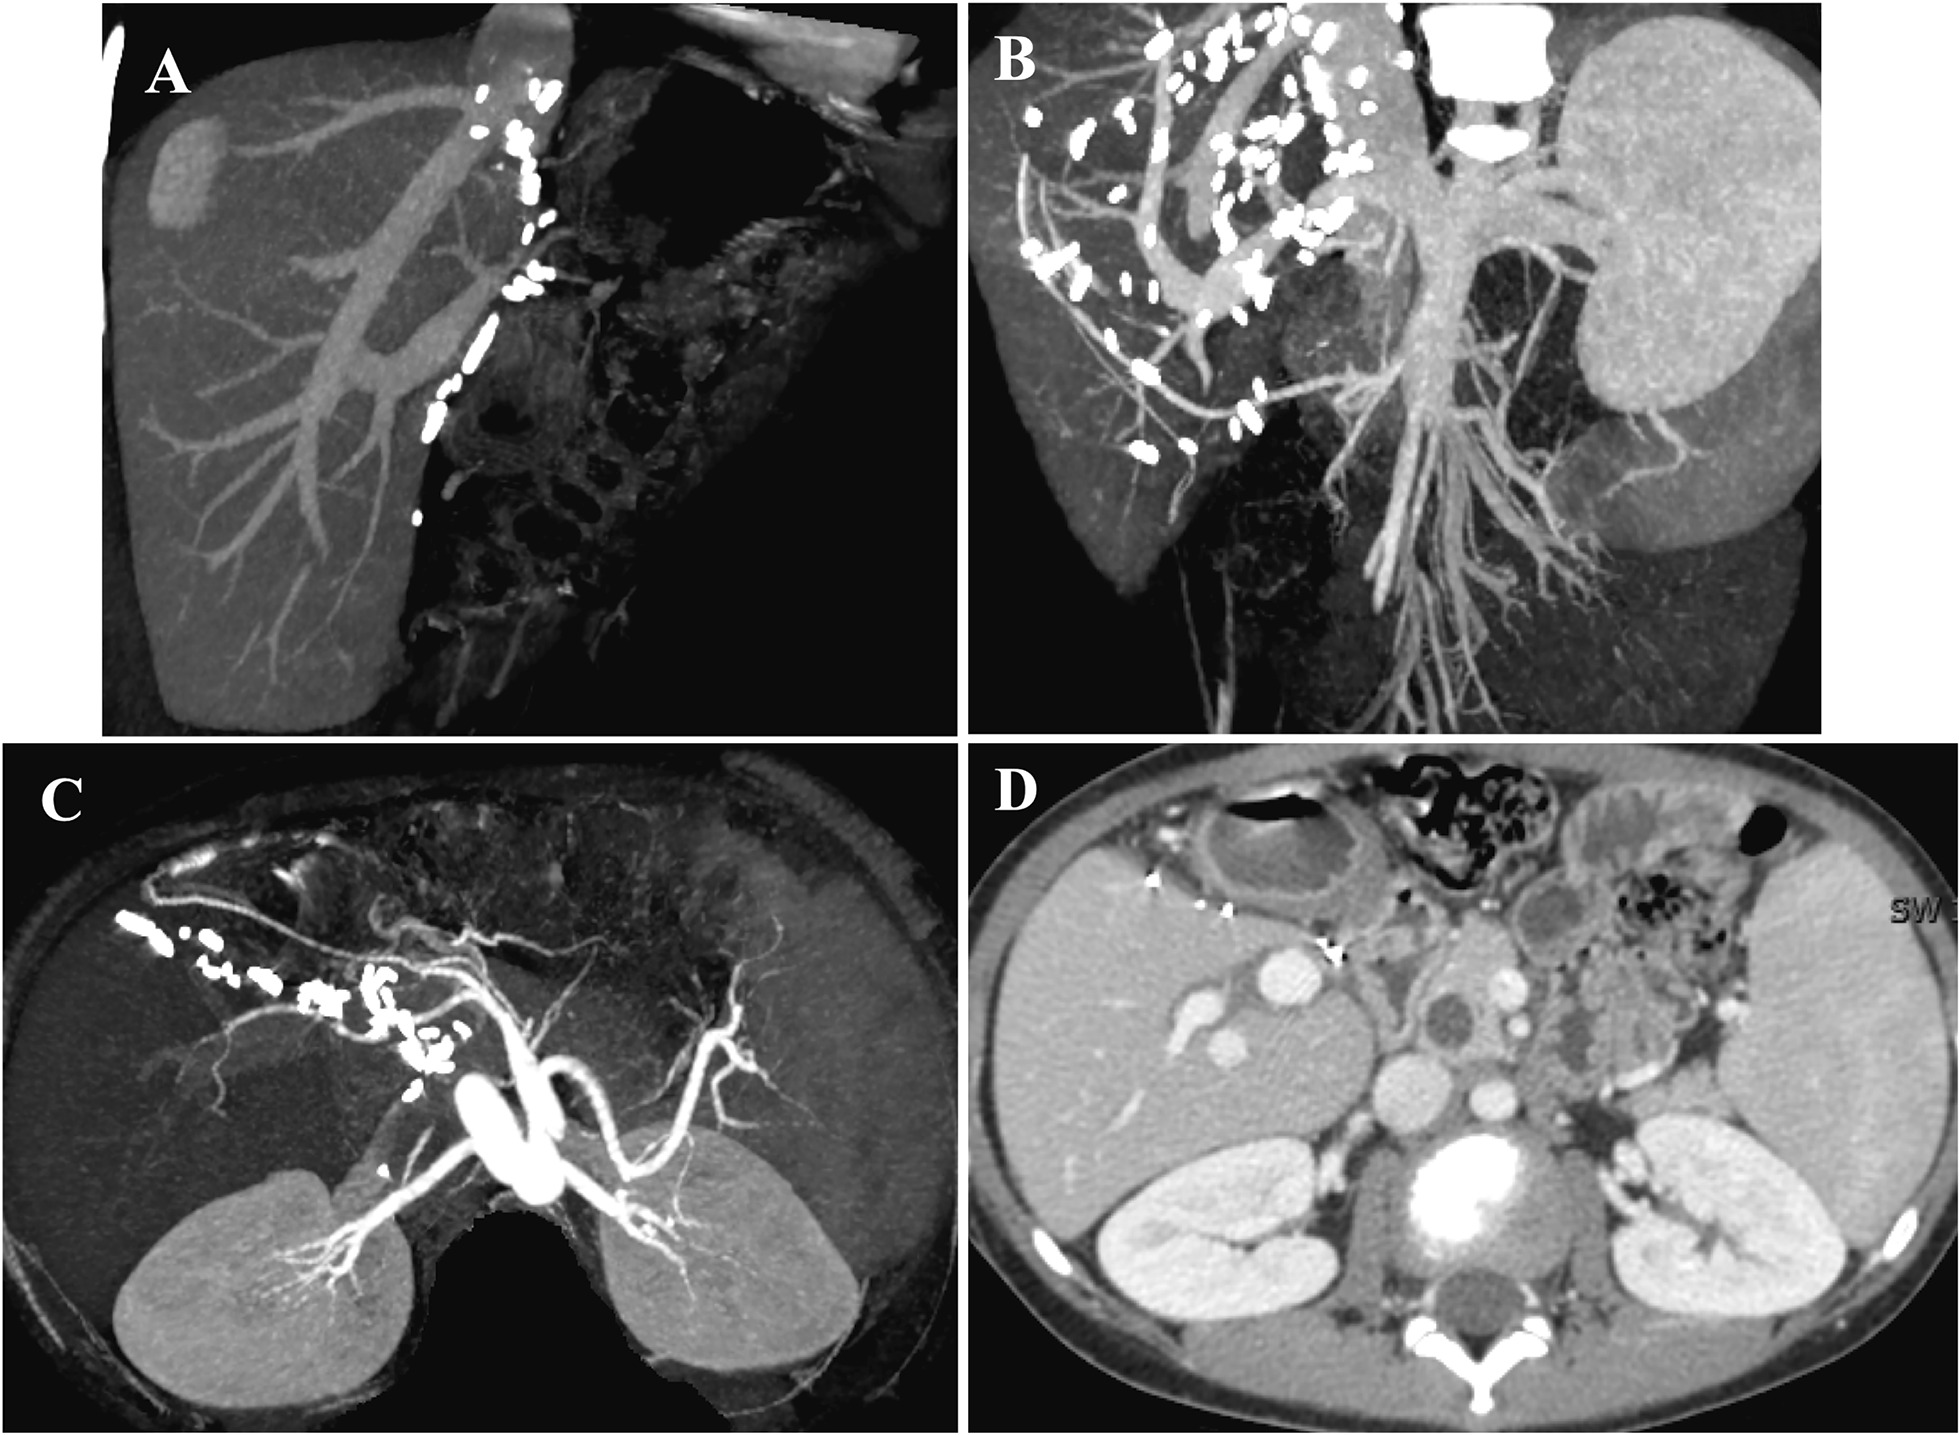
Postoperative imaging of the recipient 13 months after the transplant operation in case 1. A, B The portal and hepatic vein system. C The hepatic artery system. D The CT tomography scan showed well graft condition after the transplantation
